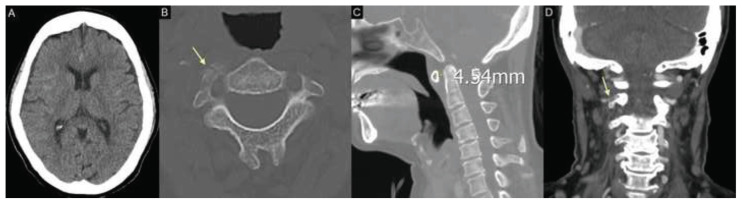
(A) Brain computed tomography (CT) demonstrating traumatic subarachnoid hemorrhage. (B) Cervical spine CT showing a fracture involving the right C2 pedicle and foraminal wall (yellow arrow). (C) Mild widening of the C1–C2 atlantodental interval (ADI: 4.54 mm), suggestive of instability. (D) CT angiography demonstrating focal irregular narrowing of the right vertebral artery (VA) at the V3 segment adjacent to the fracture site, raising suspicion of traumatic dissection (yellow arrow).
